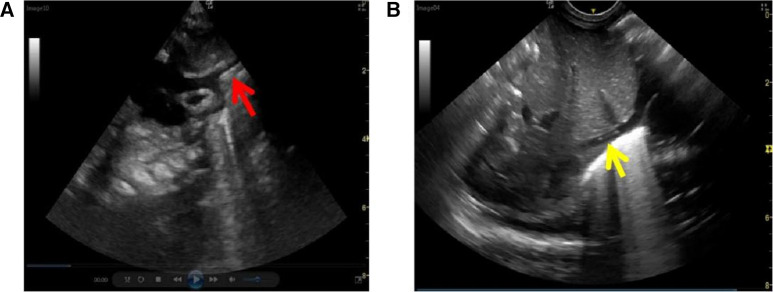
PICC ultrasonic imaging of vena cava. (A) superior vena cava (red arrow), and (B) inferior vena cava (yellow arrow).
